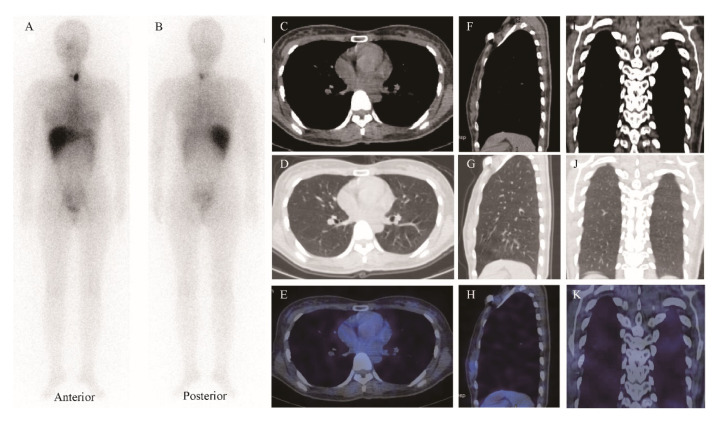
Whole-body scan ((A), anterior view; (B), posterior view) on day 9 post ^131^I administration, showing ^131^I accumulation only in the thyroid lobe remnant. SPECT/CT images of chest (C–E), transaxial; (F–H), sagittal; and (I–K), coronal), showing neither ^131^I accumulation in the chest nor pleural effusion.
